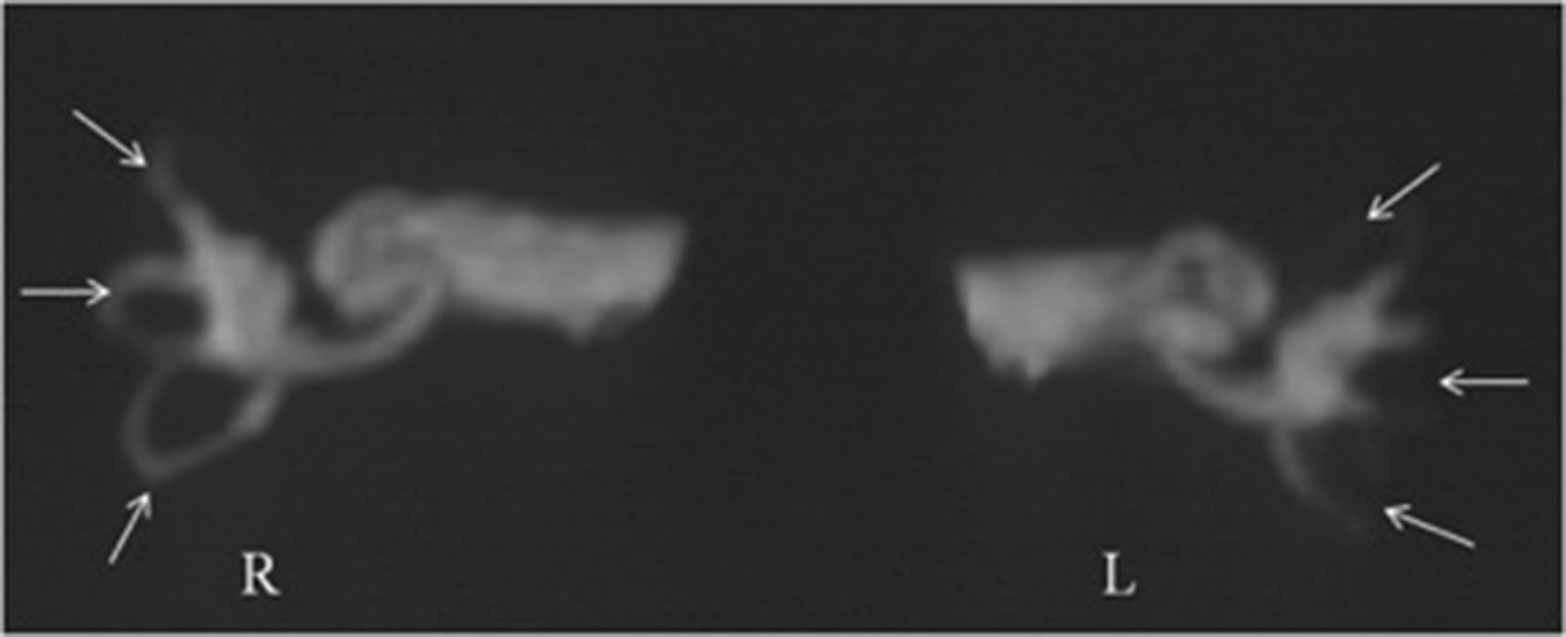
Magnetic resonance imaging of the inner ear fluid 2 years after TSCP of a 69-year-old patient with DEH of the left ear. Endolymph fluid in the position of plugging of the three semicircular canals of the left (pathological) ear contained no water (arrows), whereas endolymph fluid of the right (healthy) ear was normal. *SSC* superior semicircular canal; *HSC* horizontal semicircular canal; *PSC* posterior semicircular canal; *R* right ear; *L* left ear.
